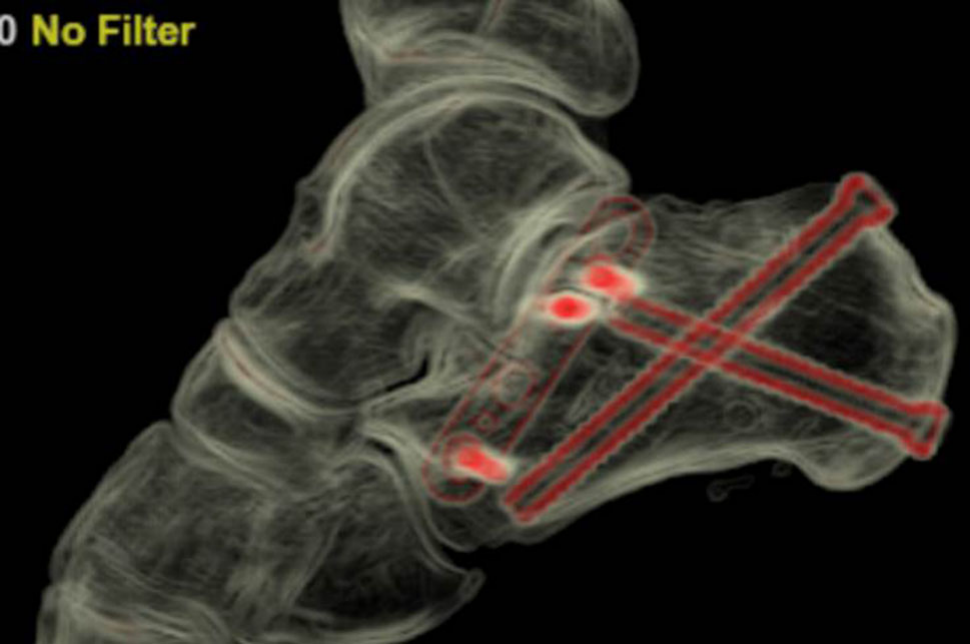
Reconstructed post-operative sagittal view from CT
